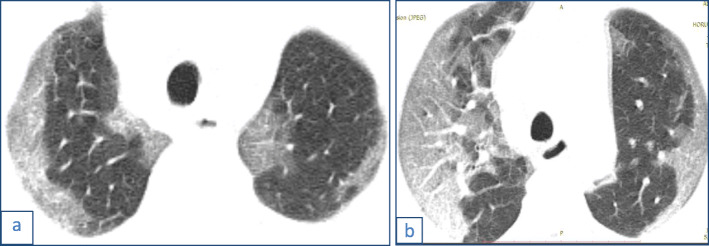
A 53-year-old male patient with COVID-19, CT chest axial images (a) and (b) show subpleural crazy paving of all lobes with vascular thickening within
